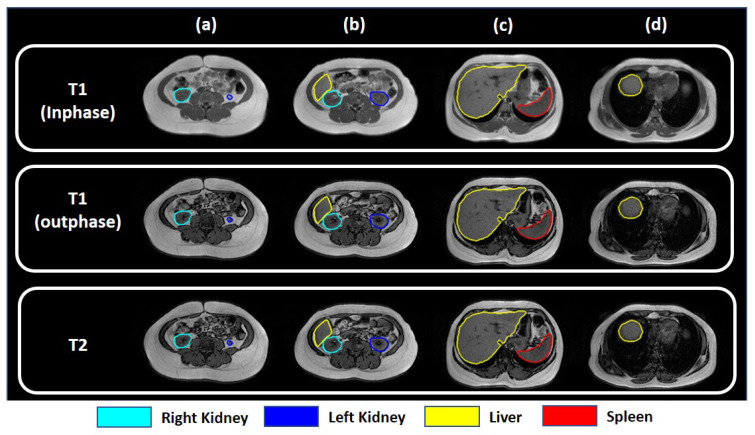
Visualization of MRI slices from (a) upper pole of the kidney, (b) inferior part of the liver, (c) middle part of the liver, and (d) superior part of the liver for different types of data available in the dataset.
